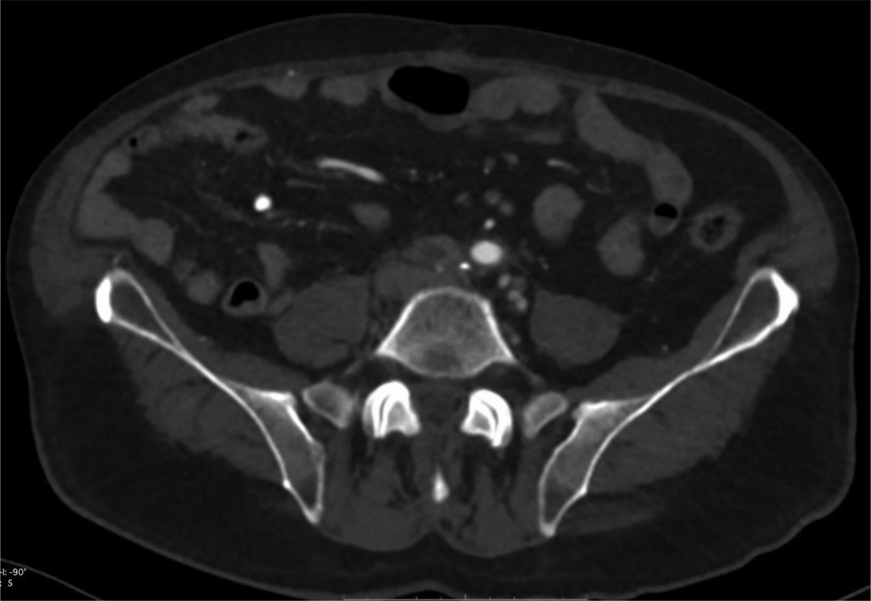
Computed tomography scan showing occlusion of the right stent.
